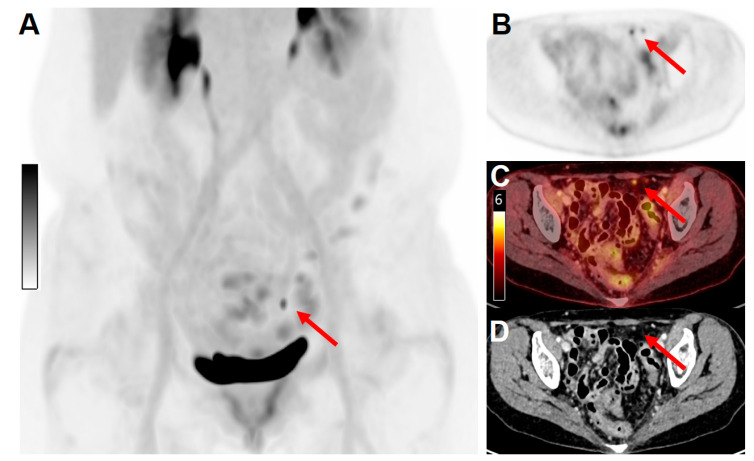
Example of the high sensitivity and spatial resolution of a long-axial field-of-view scanner. A 64 y/o female patient with cervical cancer was treated with surgery (hysterectomy and pelvic lymphadenectomy), followed by adjuvant radiation therapy, and systemic treatment with cisplatin. For response-to-treatment assessment, the patient underwent a PET/CT examination on the Biograph Vision Quadra scanner. (A) Maximum intensity projection (MIP) of images acquired 60 min after injection of 130 MBq [^18^F]FDG. (B) PET only, (C) fused PET/CT, and contrast-enhanced CT (D) images revealed the presence of two peritoneal metastatic lesions (red arrows, maximum diameter 4 mm) with clearly distinguishable [^18^F]FDG uptake (SUV_max_ 4.6).
